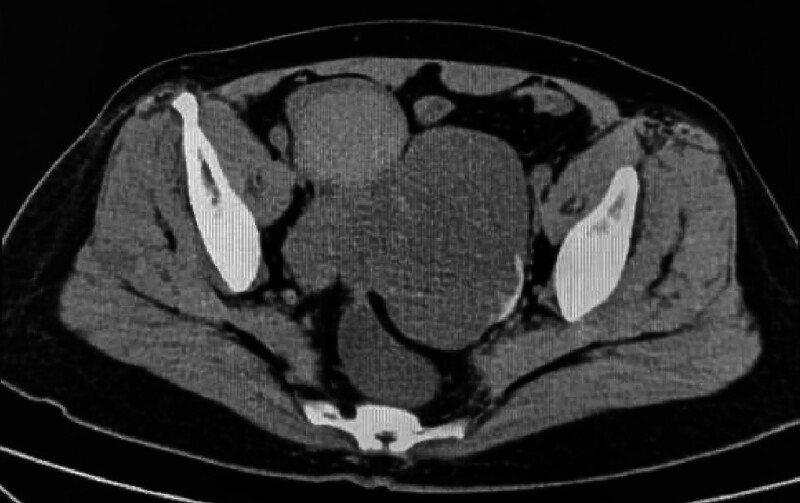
Abdominal computed tomography shows an irregular slightly low-density mass measuring 9.8 × 7.9 × 7.2 cm on the left side of the uterus.
